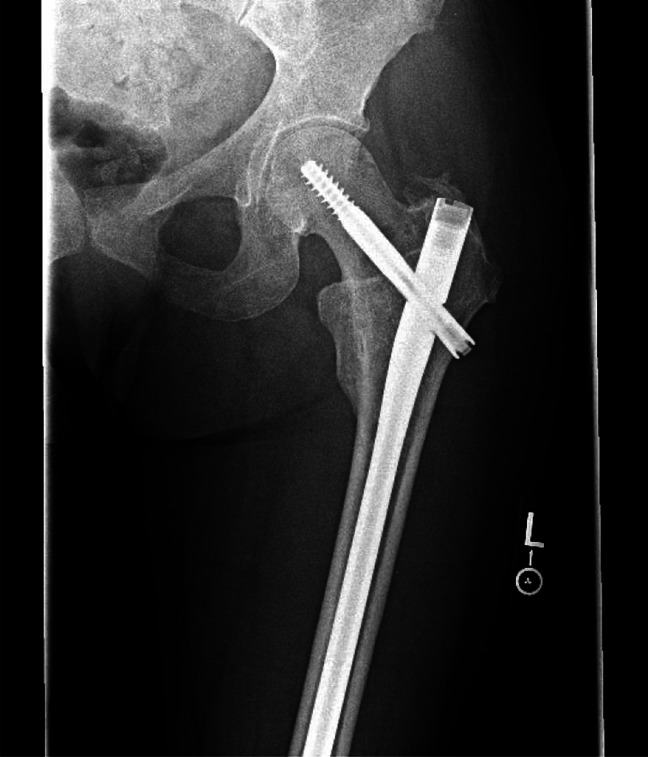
An AP radiograph of the left hip with intact implant at a 30-month postoperative visit.
